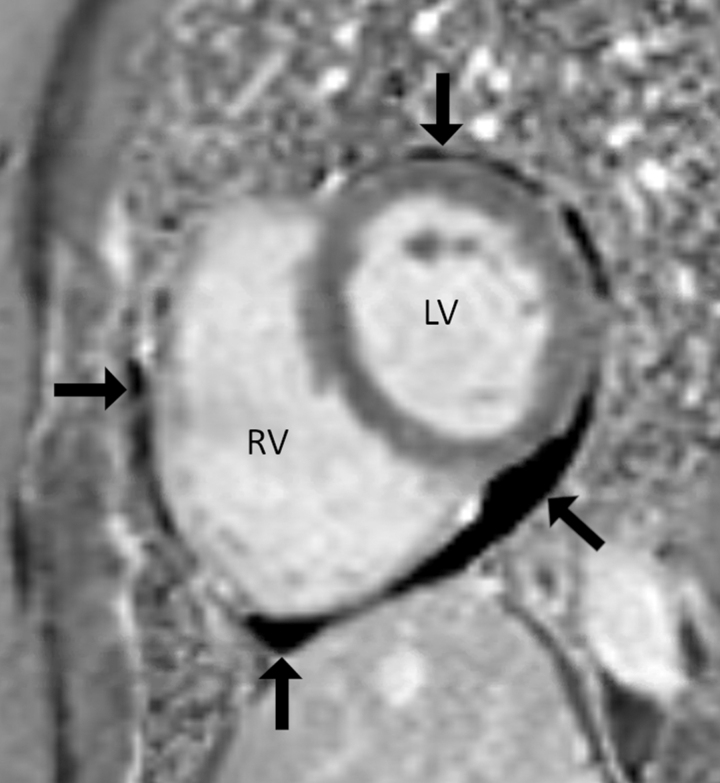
Pericardial effusion (*arrows*). Cardiac magnetic resonance image, short-axis view, and phase-sensitive inversion recovery sequence. *Arrows* indicate a hypointense signal extending circumferentially around the heart, which represents a pericardial effusion. There is no pericardial thickening in this sequence, or enhancement with gadolinium in the other sequences, which are not shown. LV, left ventricle; RV right ventricle.
